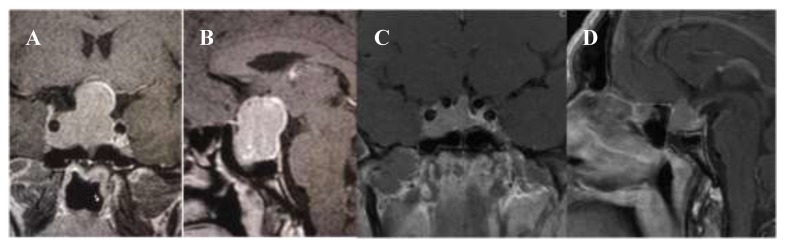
Magnetic resonance imaging showing macroadenoma (mass of 3.6 × 3.3 × 2.2 cm) in the sella turcica with right latero- and supra-sellar extension encasing the right internal carotid artery and compressing the optic chiasm: grade 4 according Knosp (A: coronal, B: sagittal plane); more recent MRI showing a decrease in the size of the macrodenoma, still extending towards the floor of the third ventricle and encasing the right cavernous sinus in the coronal plane (C); in the sagittal plane, a rather lobulated suprasella extension is shown but no evidence of stalk involvement (D). Initial laboratory testing confirmed an elevated serum IGF-1 of 988 (94-284) ng/mL, no suppression of GH after a 75 g glucose load (7 ng/mL), mild hyperprolactinemia and hypogonadism.
